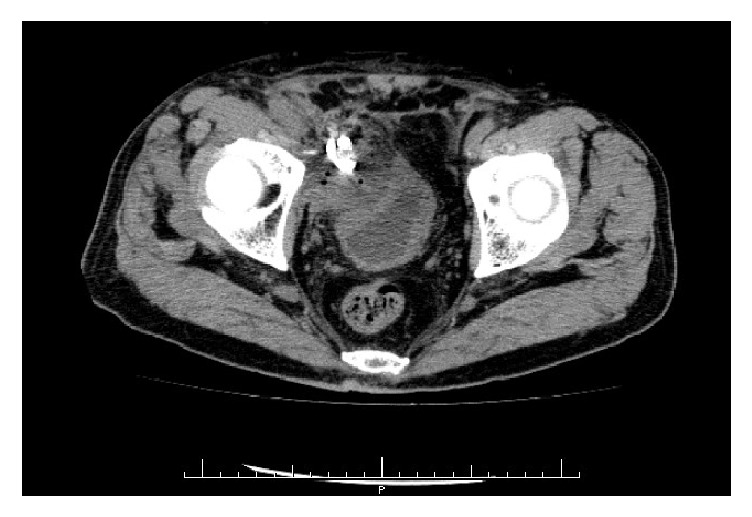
CT scan shows reduction of the lymphocele few days after clamping.
